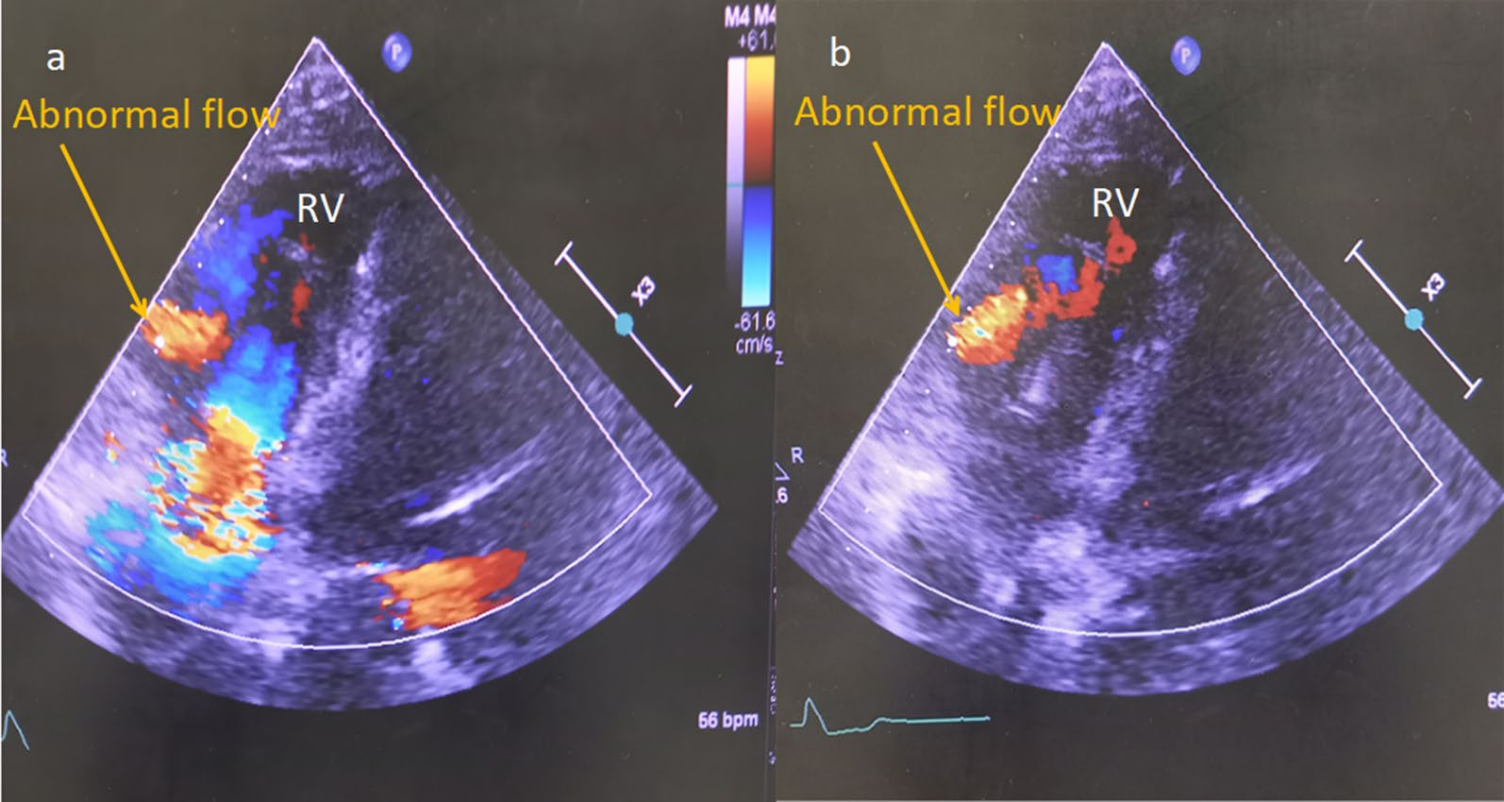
Preoperative transthoracic echocardiography. a Abnormal flow (arrow) into the RV observed in the four-chamber view of the systolic phase. b Abnormal flow (arrow) into the RV in the diastolic phase. RV, right ventricle
